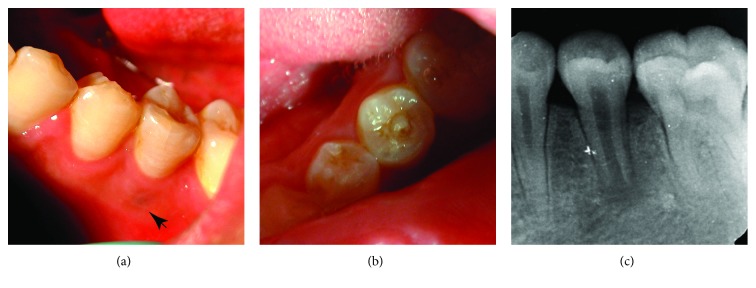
Clinical photograph and periapical radiograph of this case. (a) The clinical photograph shows a violet-blue patch with a diameter of 2 mm in the buccal gingiva of tooth #20. (b) The clinical photograph shows an attrited abnormal center cusp at the center of the occlusal surface of tooth #20. (c) The periapical radiograph shows a radiolucent lesion at the periapical area of tooth #20 with a wide open apex.
